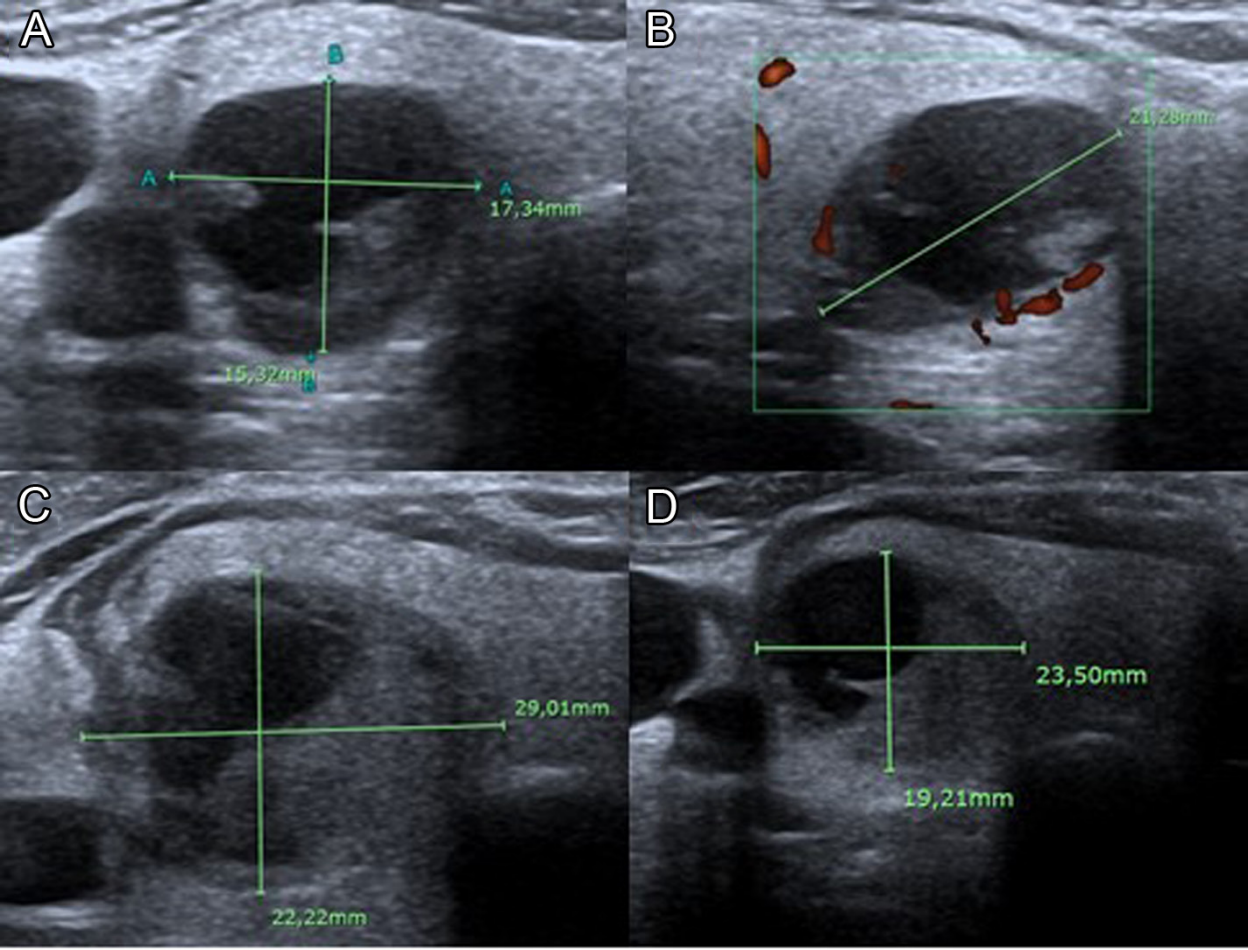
(A) Transverse and anteroposterior and (B) longitudinal diameters of the intrathyroid nodule in the ultrasound (US) images of the neck performed at the time of US-guided fine-needle aspiration (FNA). Image of the nodule 3 (C) and 10 days (D) after FNA.
